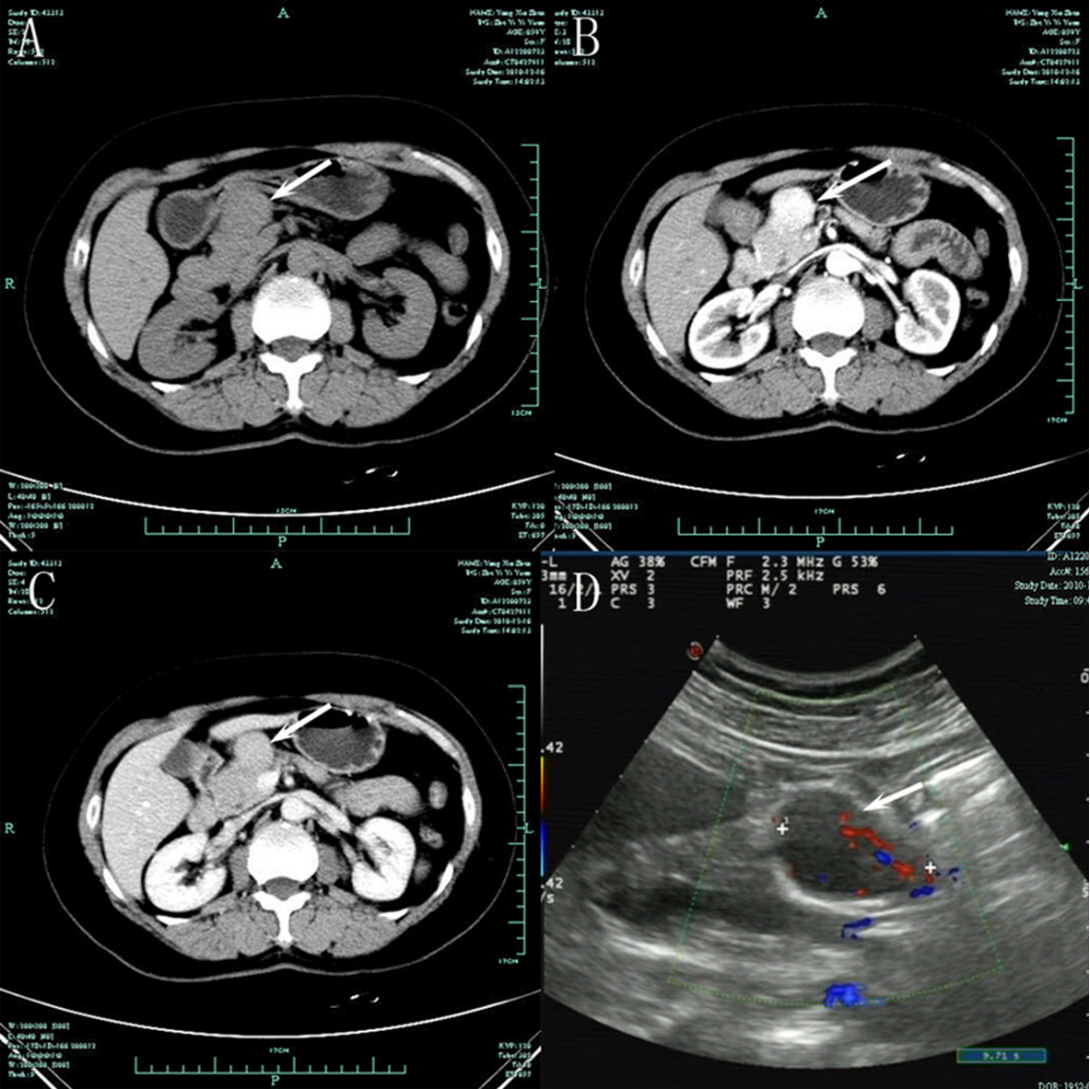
Image findings of case 2. Case 2: Axial contrast-enhanced CT image of the abdomen depicts a 4 × 2.7 cm moderately enhancing mass (arrows) at pancreatic neck. Axial unenhanced (A), arterial phase (B), and delayed phase (C) CT images of the abdomen and ultrasonography (D).
